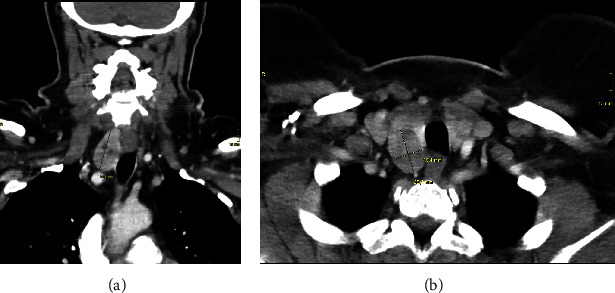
Preoperative computed tomography scan of the neck. (A) Computed tomography scan with the right thyroid lobe enlarged dorsally but without evidence of cervical lymph node metastasis. (B) The right thyroid lobe is increased to 18 mm × 25 mm × 33 mm in the dorsal part (dimensions are highlighted with solid lines).
